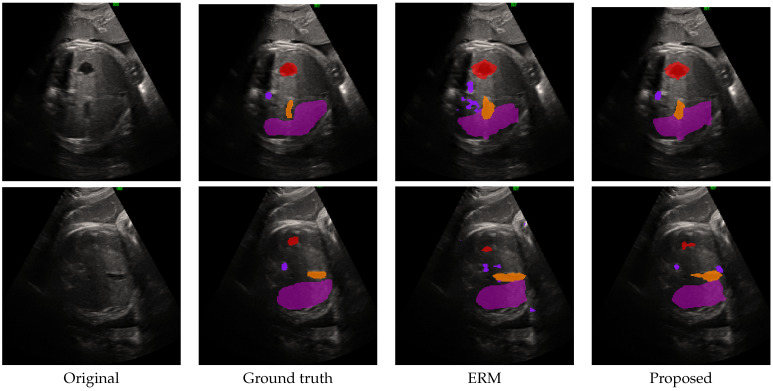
Example of segmentation of fetal abdominal structures in ultrasound imaging. Each row represents a case. With respect to annotations, with magenta is the “stomach”, orange is the “liver”, red is the “artery”, while with violet is the “vein”.
